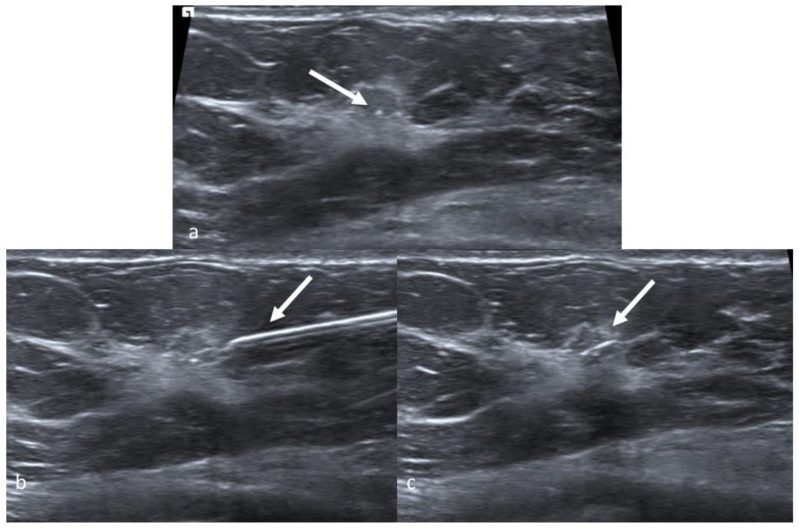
Magseed positioning in a 49-year-old woman with ductal carcinoma in situ. Ultrasound images of the right upper outer quadrant. Biopsy marker is visible in the lesion (a, arrow). Magseed magnetic marker is placed under ultrasound guidance (b, arrow shows the needle). Magseed^®^ marker is clearly seen in the lesion (c, arrow).
